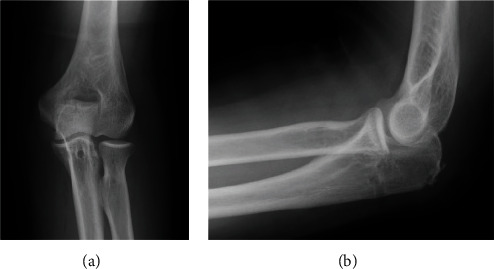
Plain radiography images of the left elbow after the osteosynthesis. Postoperative plain radiography images demonstrated that the avulsion fracture fragments were reduced (a, b).
